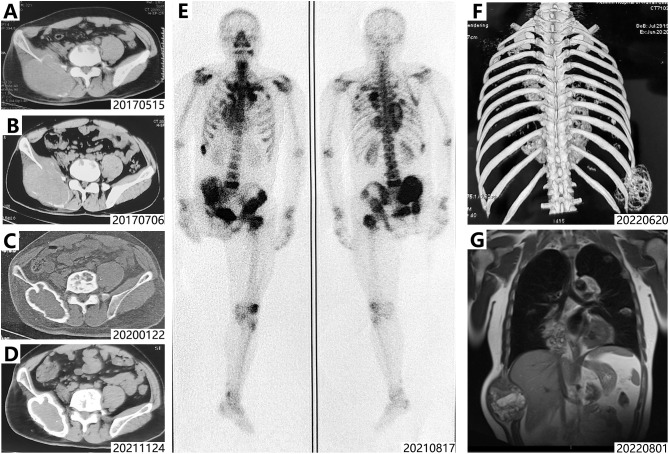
Imaging of pelvic and rib lesions and bone scintigraphy. (A, B) Slowed progression and fine peripheral rim calcification were found in pelvic lesions after initial denosumab therapy. (C, D) Reduction in size and development of distinct rim calcification were observed in pelvic lesions after denosumab treatment; no progression was noted on long-term follow-up. (E) Bone scintigraphy of August 2021 revealed multiple osteolytic lesions, consistent with multicentric giant cell tumor of bone. (F) CT scan of the ribs in June. 2022 reveals a large mass (approximately 9 cm × 8.5 cm × 10 cm) at the axillary segment of the right tenth rib. (G) MRI reveals a heterogeneous signal intensity lesion involving the right tenth rib, predominantly hyperintense on T2-weighted images. The lesion displaces the right lobe of the liver medially and protrudes outward from the chest wall.
